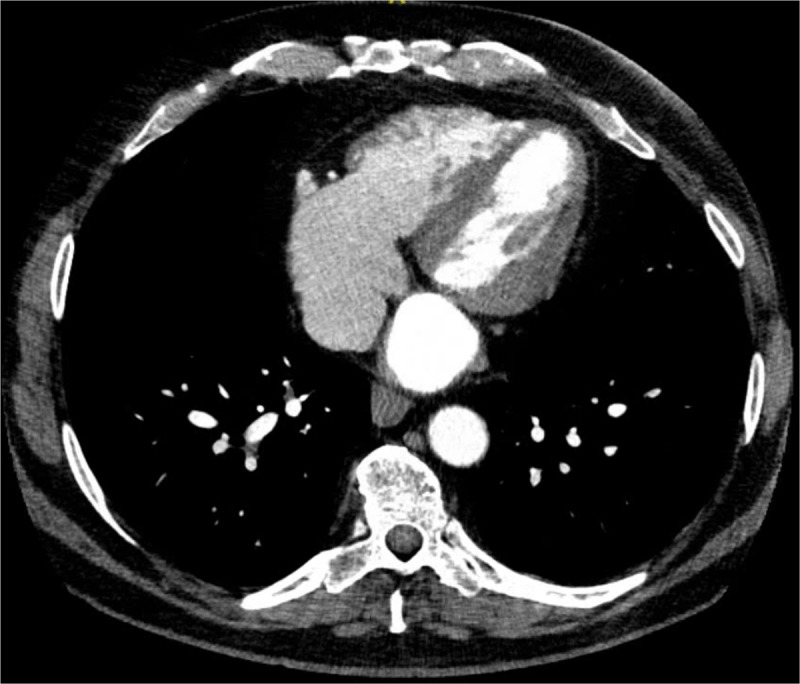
Computed tomography done prior to the atrial fibrillation ablation did not show a pseudoaneurysm.
